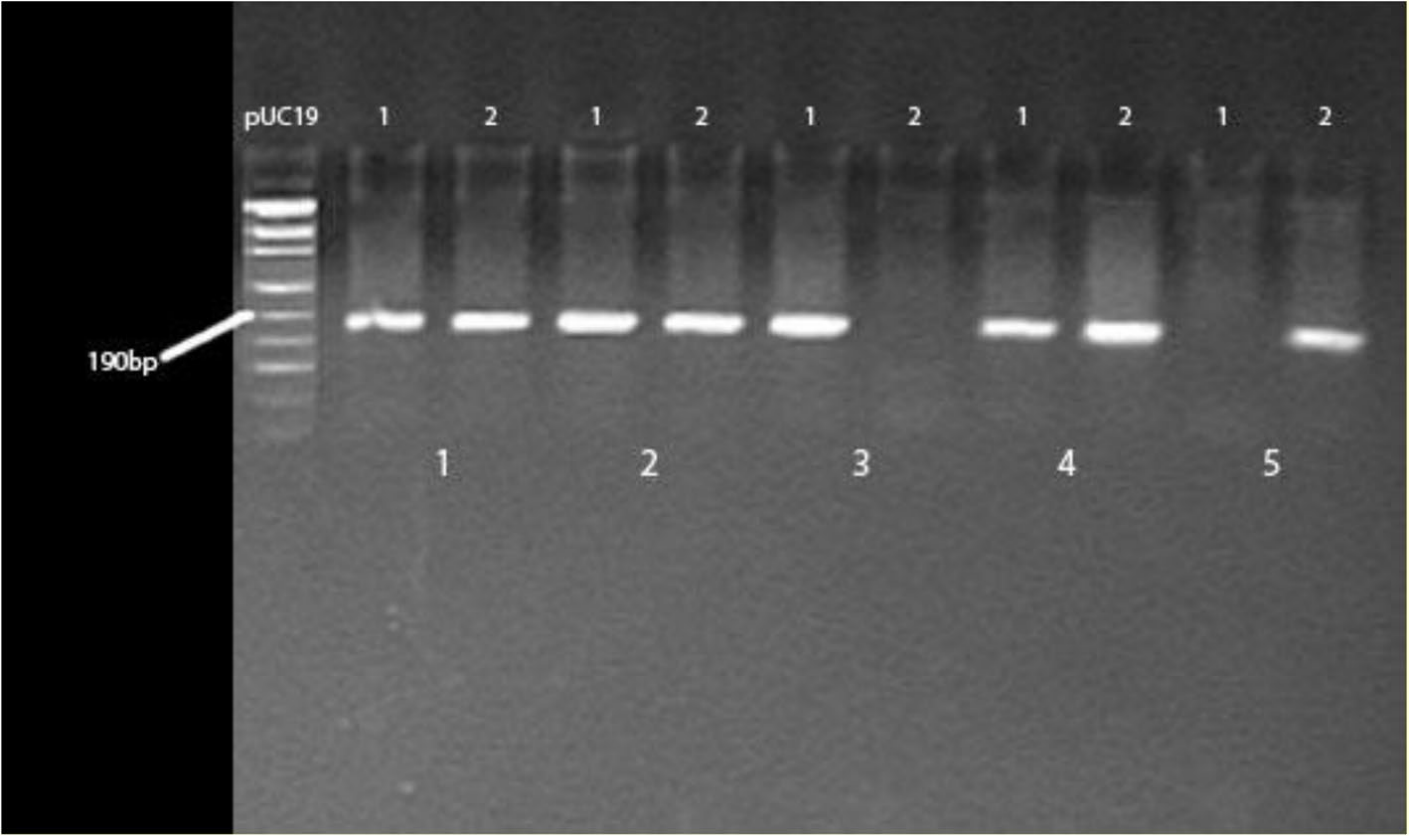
LDLR* rs688 (C/T) polymorphism in the allele-specific PCR assay. A 191 bp DNA fragment indicates the presence of the allele in the sample. In each pair of reactions sample 1 represents C allele and sample 2 represents T allele. Legend: pUC19 DNA/Msp I (Hpa II) Marker. Genotypes: pair 1, 2, 4 - CT, pair 3 - CC, pair 5 - TT
